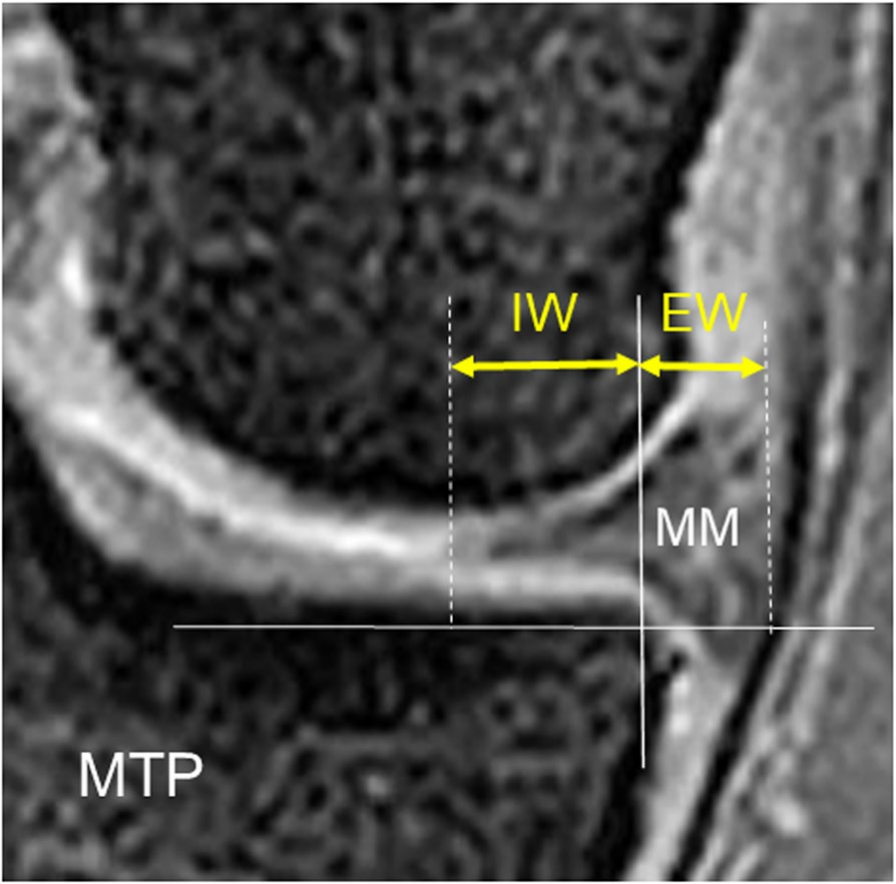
Measurement of the location of the medial meniscus (MM) on the coronal image of the MRI. A vertical line intersecting the peripheral margin of the medial tibial plateau (MTP) was drawn. Then, the second and third lines, which were parallel to the first line, were drawn at the outer and inner margins of the meniscus, respectively. The distance between the first and second lines, which was defined as “Extrusion width (EW)”, and the distance between the first and third lines, which was defined as “Inner width (IW)”, were measured. Each width was quantified in millimeters
